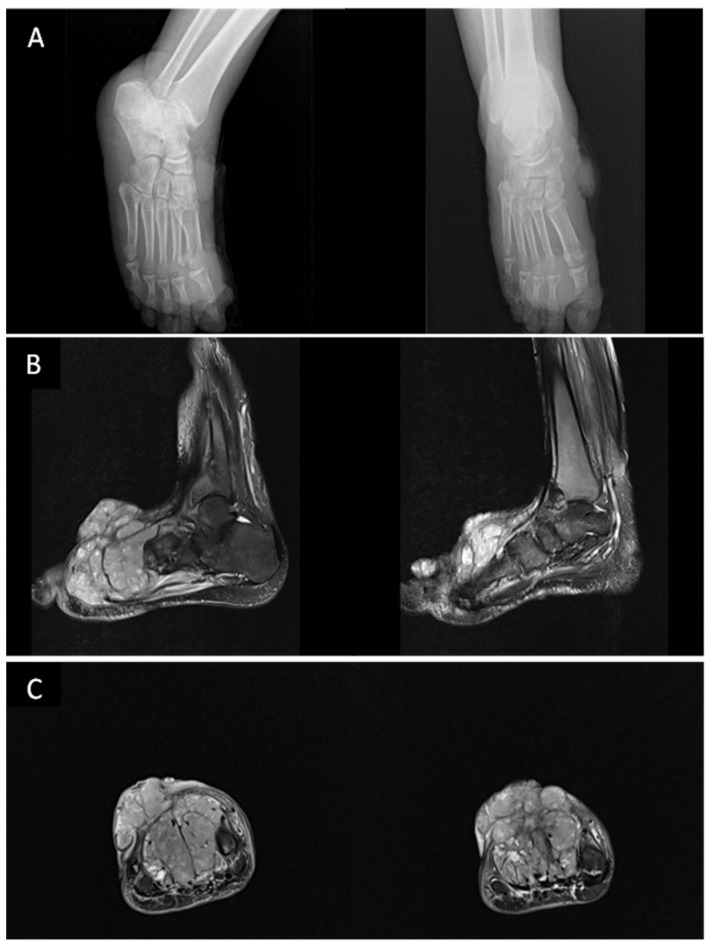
Imaging of the patient described in Figure 1. (A) Plain radiograph in the anteroposterior and lateral projection of the right foot showing lobulated soft tissue swelling involving the anterior and medial malleolus aspect without any associated periosteal reaction of the adjacent bones. (November 2015). (B,C) Magnetic resonance imaging (MRI) (Sagittal T2 FS images (B) Coronal T2 FS (C)) shows multiple enhanced exophytic cutaneous and subcutaneous masses of the right foot with osseous involvement of underlying structures (the main lesion overlying the second, third, and fourth metatarsal bones, lobulated dorsal mass adjacent to the middle phalange of the second toe) associated with a local invasion of the extensor tendons and close contact to flexor tendons (February 2019).
